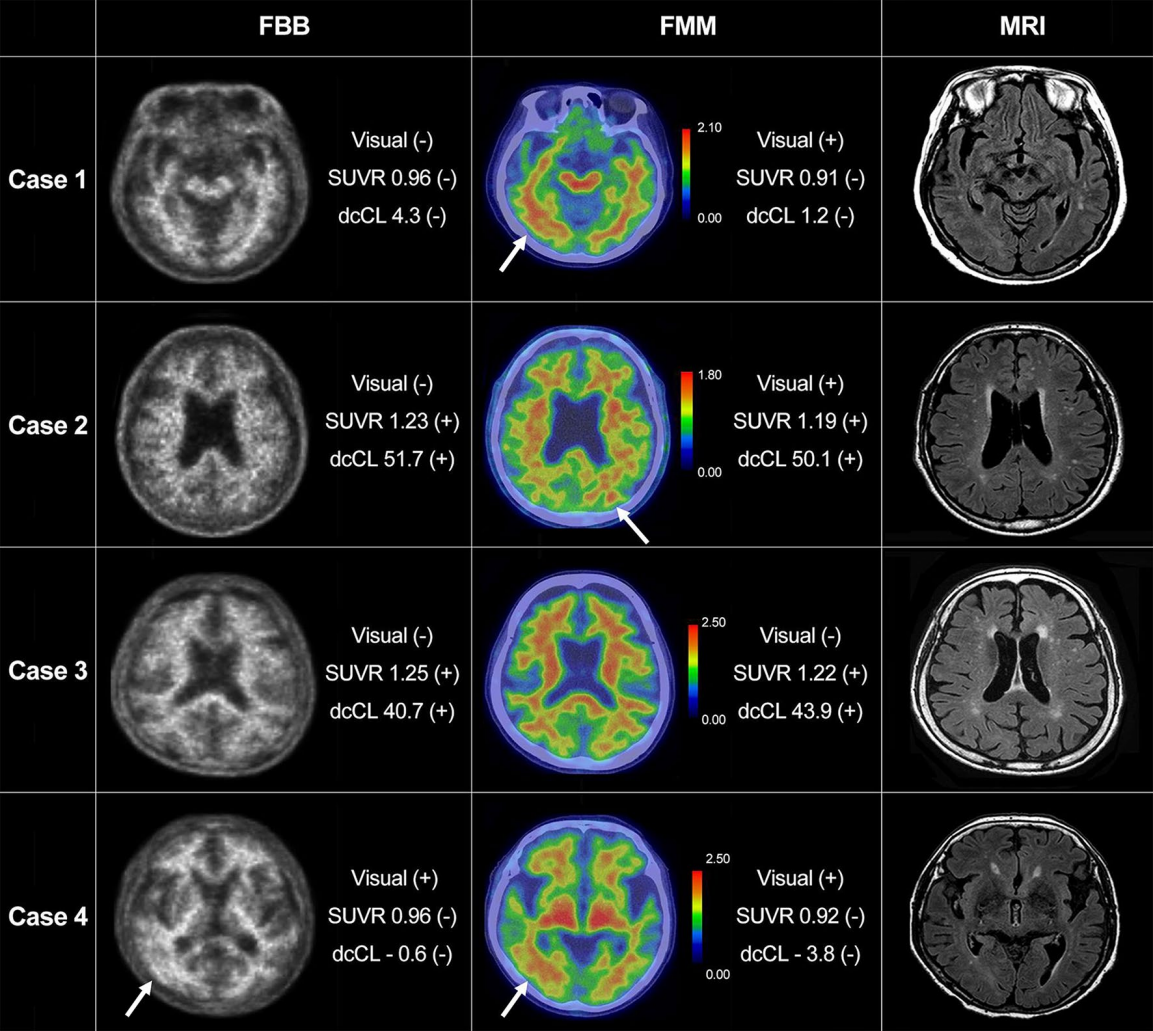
FBB and FMM uptake in participants. Four representative cases of FBB and FMM PET and FLAIR MRI are shown. Case 1 represents the participant who was FBB-negative but FMM-positive based on visual assessment although SUVR and dcCL positivity were both negative. Case 2 represents the participant who was FBB-negative but FMM-positive based on visual assessment although SUVR and dcCL positivity were both positive. Case 3 represents the participant whose visual assessment was negative but SUVR and dcCL positivity were both positive for FBB and FMM. Case 4 represents the participant who was positive based on visual assessment but SUVR and dcCL positivity were both negative for FBB and FMM. The Scale bar indicates standardized uptake values (SUVs). The arrow indicates focal uptake of FMM in the cortex. Abbreviations: FBB, ^18^F-florbetaben, FMM, ^18^F-flutemetamol; FLAIR, fluid-attenuated inversion recovery; SUVR, standardized uptake value ratio; dcCL, the Centiloid (CL) units using the direct comparison of FBB-FMM CL method.
